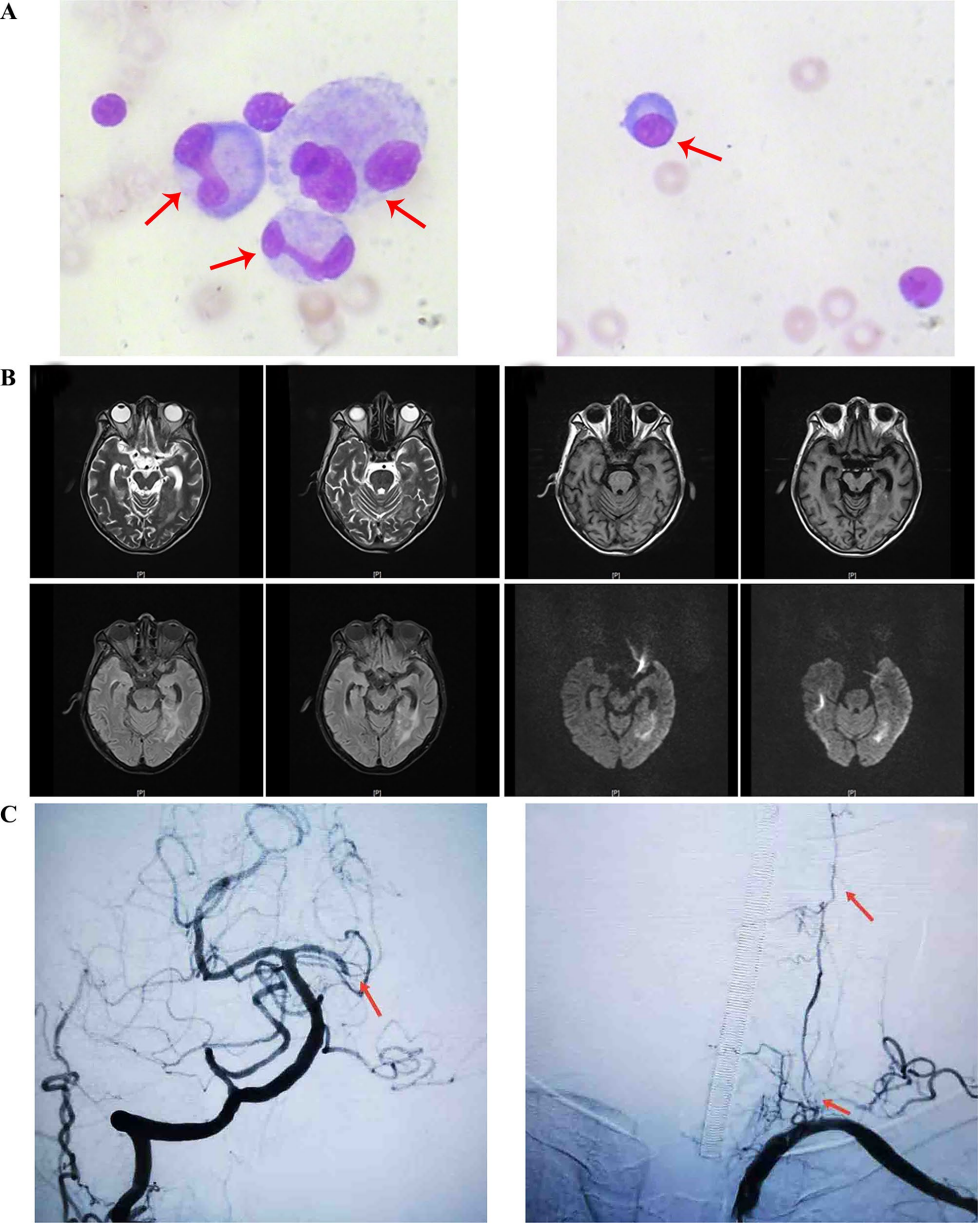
Results of imaging and cerebrospinal fluid cytology examinations. (a) Image of cerebrospinal fluid cytology examination. Active monocytes and plasmacytes from left to right were indicated by the red arrow. (b) Brain MRI results. These images were in pairs from left to right and from top to bottom, and represented T1, T2, Flaire, and dwi, respectively. (c) Cerebral angiography examination. Abnormal signals were indicated by the red arrow
